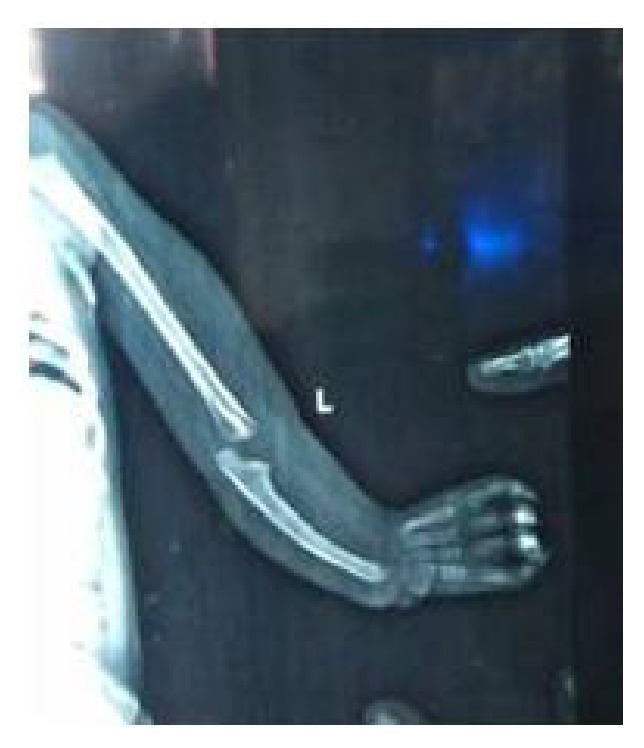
X-rays left hand: absent radius with absent thumb bone.
